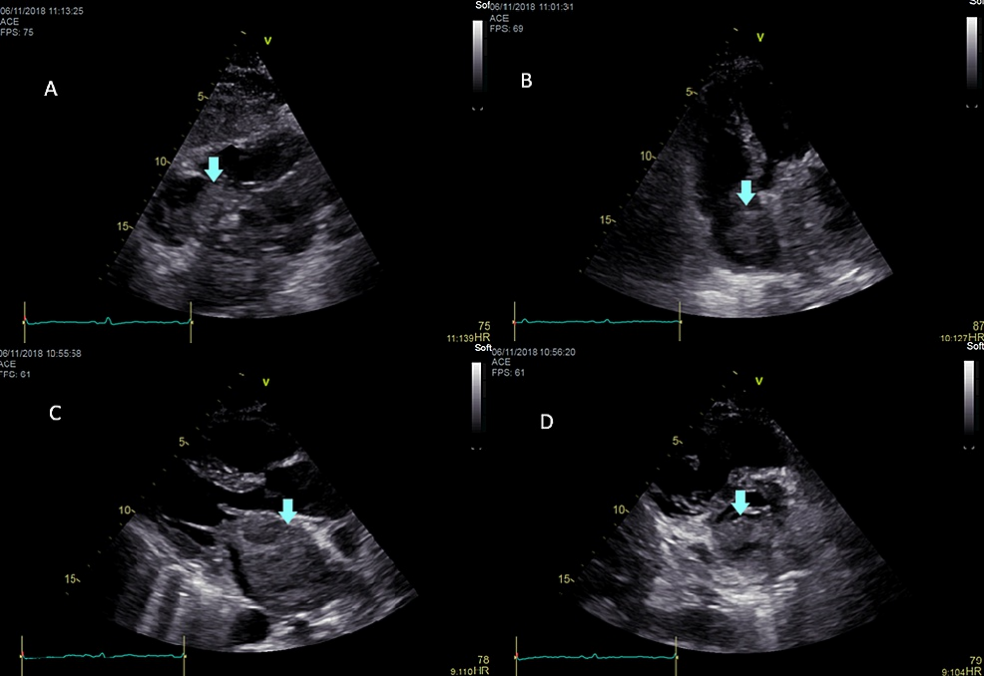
Transthoracic echocardiogram of the left atrium showing a voluminous mass infiltrating the atrial septum and extending into the right atrium (blue arrows). (A) Subcostal four-chamber view, (B) apical four-chamber view, (C) parasternal short-axis view, and (D) parasternal long-axis view.
